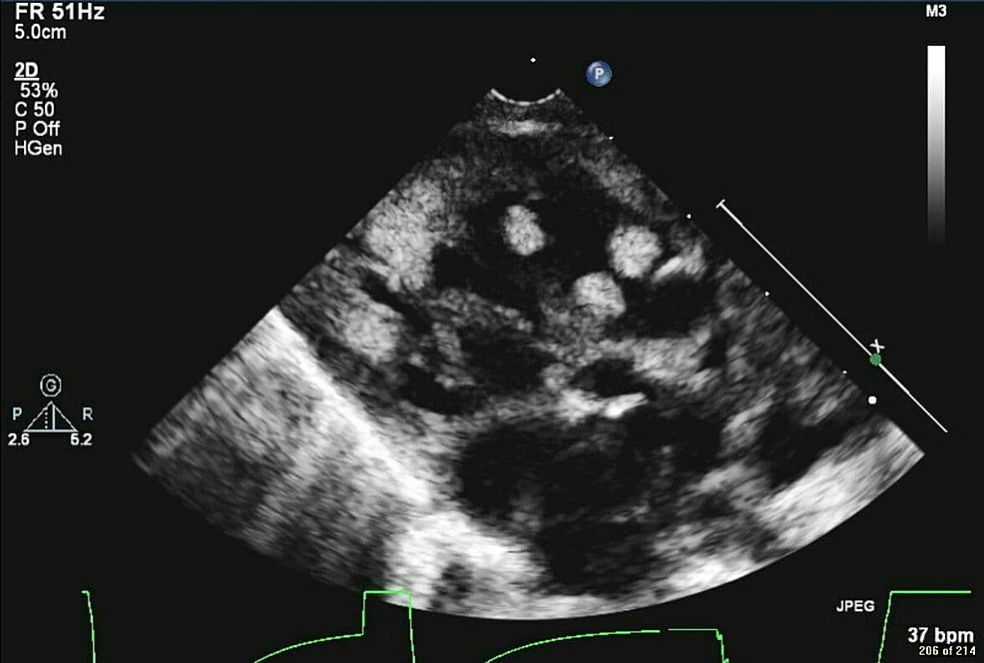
Echocardiography shows multiple echogenic masses (five at least seen in the ventricle and the largest is 9x8)
